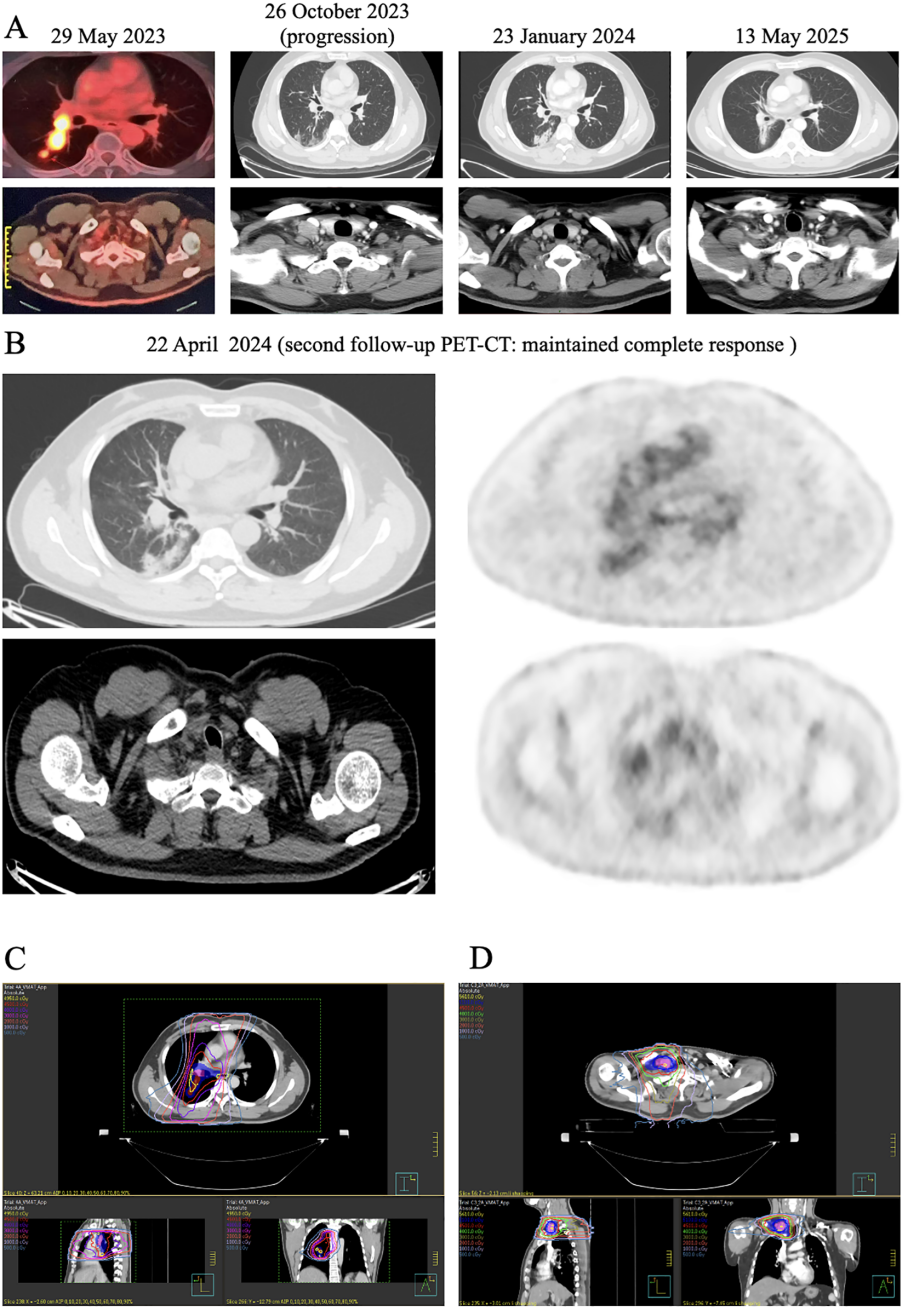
Imaging and RT plans of the patient. (A) PET-CT and CT images of the patient at four different time points; (B) PET-CT scan of the patient taken on April 22, 2024; (C) Chest RT plan; (D) Supraclavicular lymph node RT plan. Abbreviation: CT, computed tomography; PET-CT, positron emission tomography-computed tomography; RT, radiotherapy.
